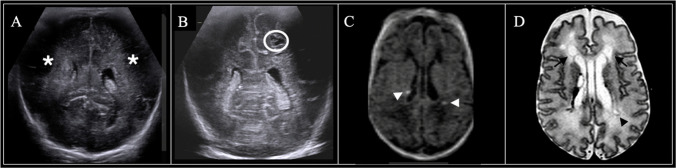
Neuroimaging in a female infant born at 29 weeks and 4 days with severe periventricular and subcortical cystic white matter injury. (A) 7-DOL coronal-plane head ultrasound demonstrated diffusely increased echogenicity equal to or brighter than choroid plexus (*asterisk*, Miller score 4); (B) 30-DOL coronal-plane head ultrasound demonstrated cavitary cystic lesions in the white matter (*white circles*, Miller score 5). Term-equivalent age MRI showed (C) greater than 6 foci of increased signal intensity in the white matter on axial T1-weighted imaging (*white arrowheads*, only a subset are marked for clarity) and (D) extensive periventricular and subcortical cysts (*black arrow*, only a subset are marked for clarity) and ventriculomegaly with decreased white matter volume. This patient also had grade II germinal matrix hemorrhage on head ultrasound (not shown), which evolved into germinolytic cysts on the term-equivalent age MRI. The Martinez-Biarge score was 4
